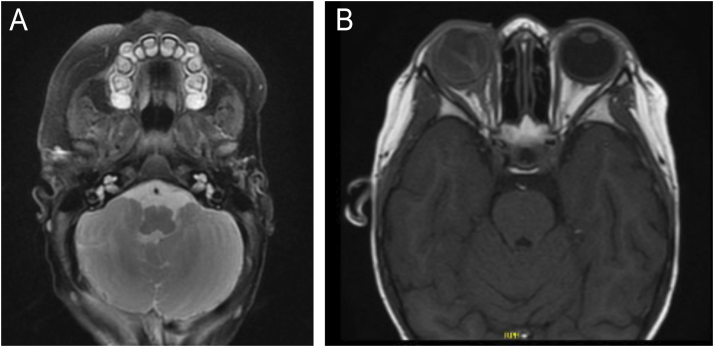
(A) MRI showing hypoplasia of the right cerebellum (case 1). (B) MRI showing right retinoblastoma (case 2).
